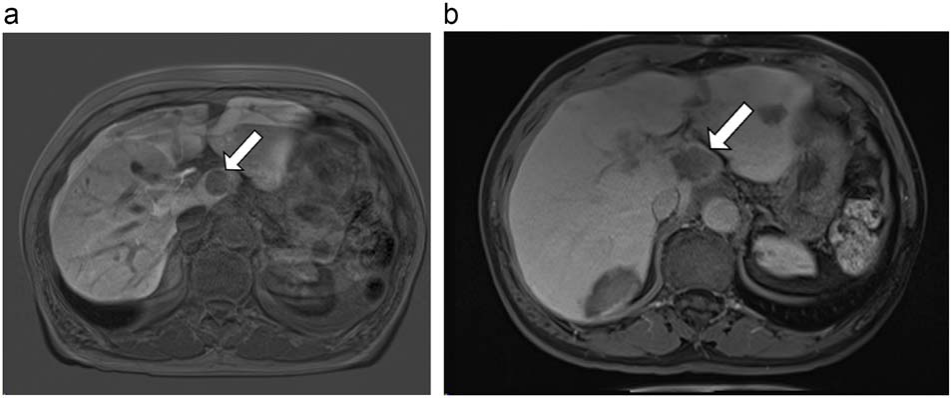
(a) Pre-ablation MRI demonstrating 28 mm caudate lesion. (b) Post-ablation MRI (16 days postoperatively) demonstrating 37.3 mm complete ablation of caudate lesion.
